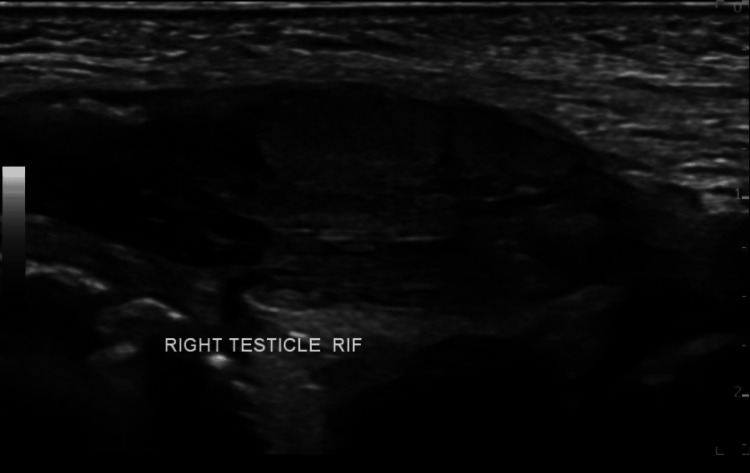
Ultrasound image showing the right testis in the right iliac fossa The scan demonstrates an ovoid, homogeneously hypoechoic structure consistent with testicular tissue, located intra-abdominally. This is indicative of an undescended right testis. RIF: right iliac fossa
